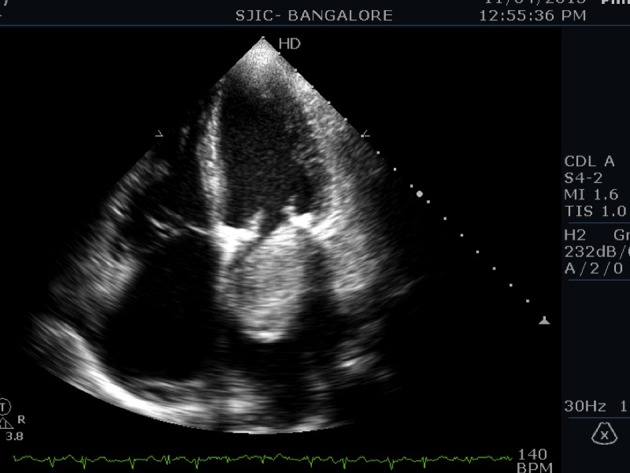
Apical four-chamber view showing a large myxoma obstructing mitral valve in diastole.
